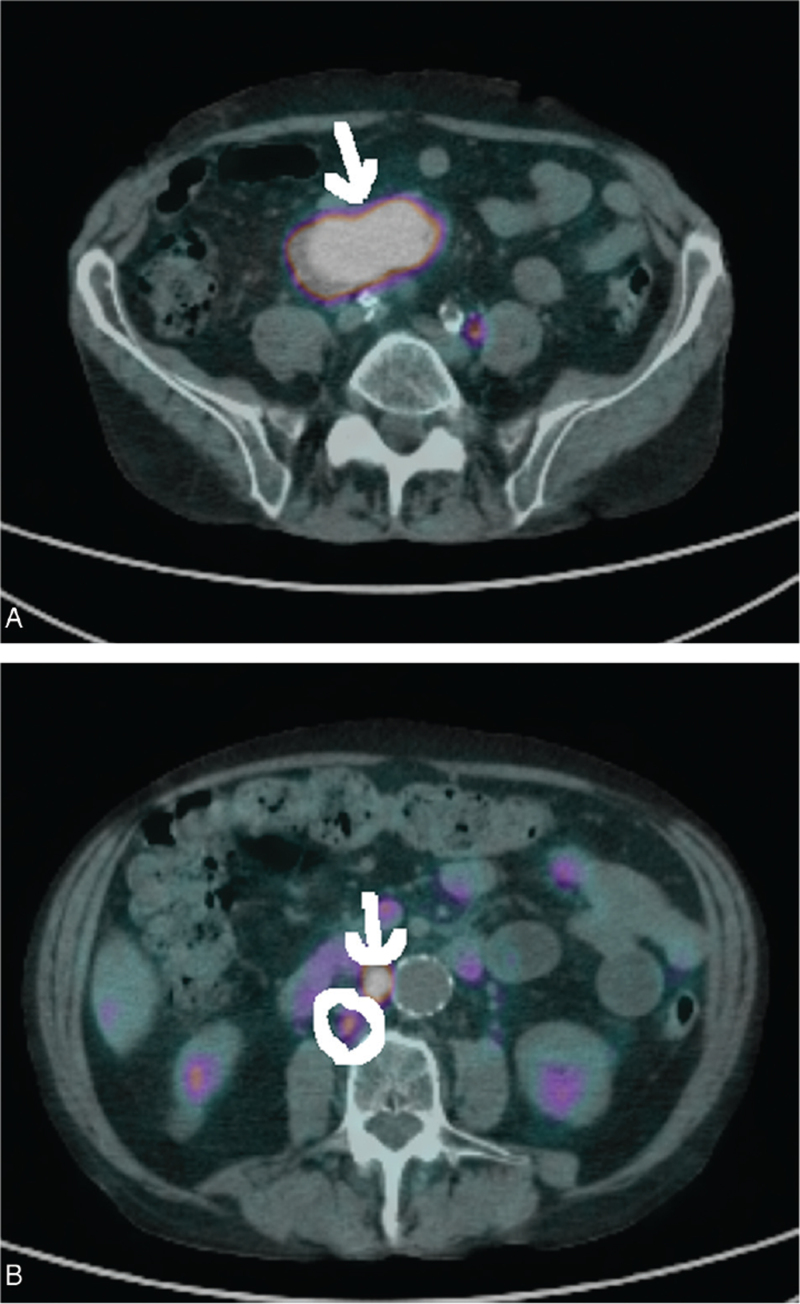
(A) Ga PET DOTATOC mesenteric tumour (arrow). (B) Ga PET DOTATOC subrenal retrocaval (circle) and interaortocaval lymph node (arrow): lymph nodes with pathologic SUV. Ga-PET = Gallium-positron emission tomography, SUV = standardized uptake value.
